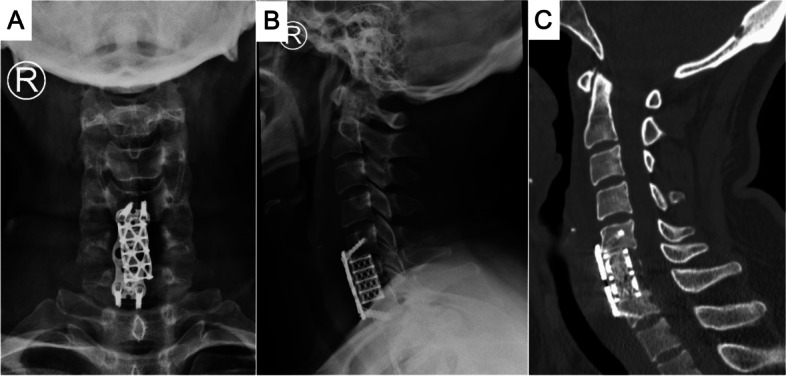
Postoperative images. A and B cervical spine plain radiography (taken on postoperative day 2); A anterior-posterior view; B lateral view; C CT scan of the cervical spine (taken on postoperative day 4), sagittal cut
